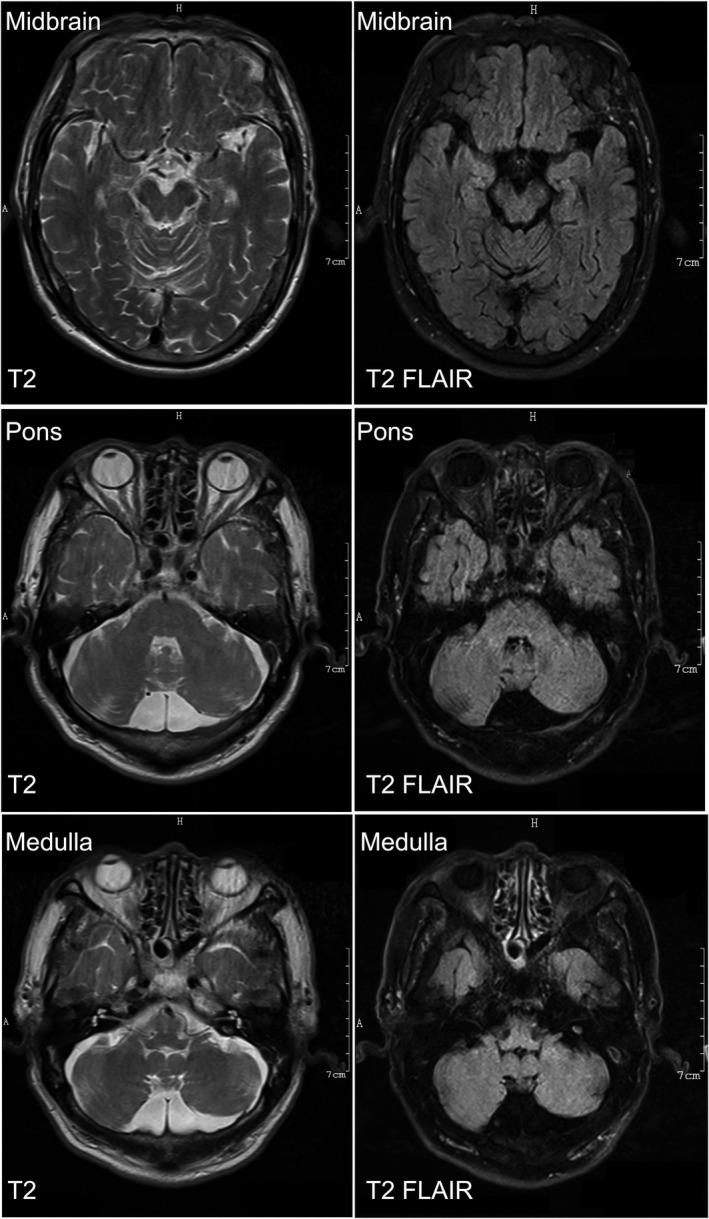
No abnormality found in brainstem (midbrain, pons, and medulla) on MR axial T2 and T2‐weighted‐Fluid‐Attenuated Inversion Recovery (T2‐FLAIR)
